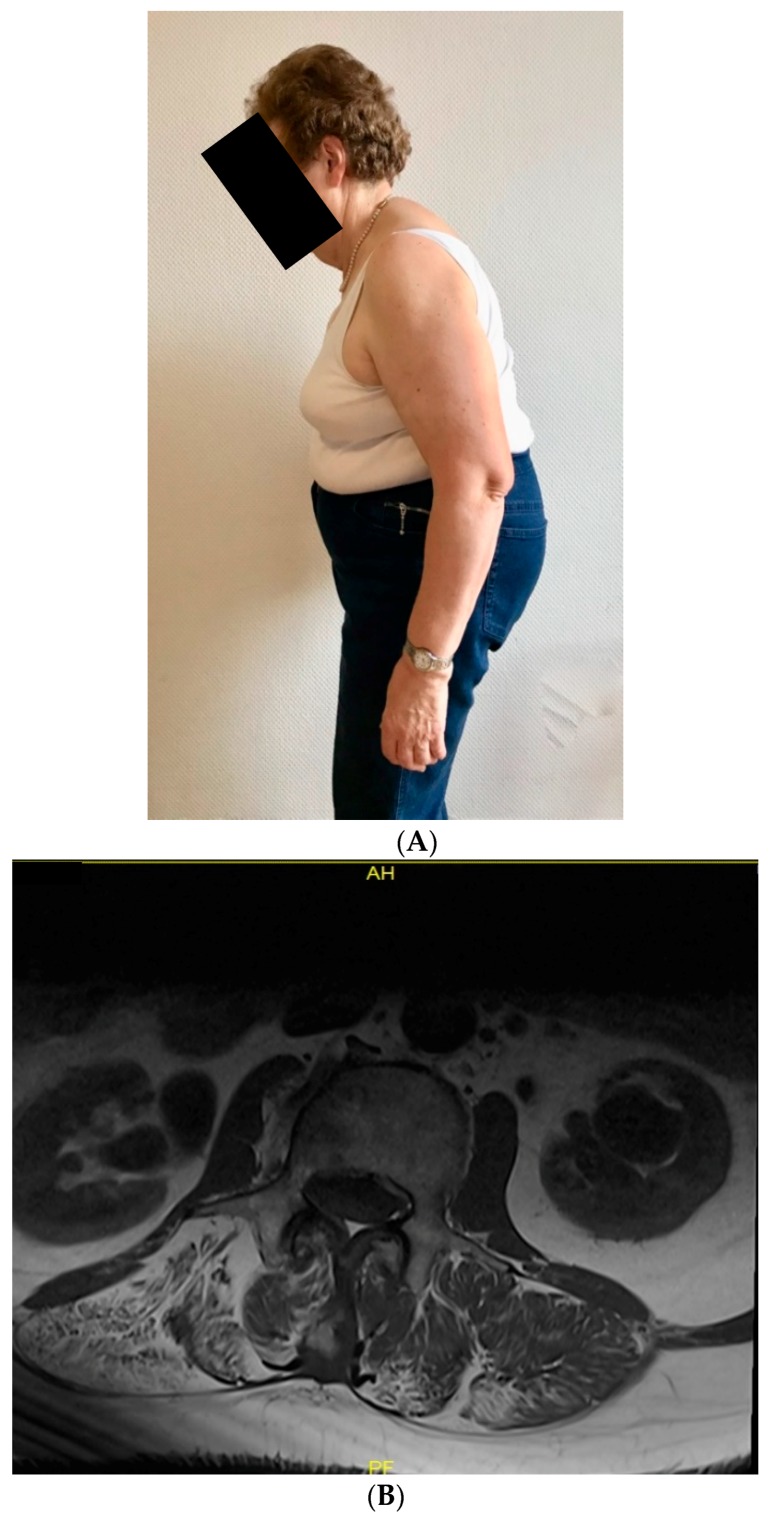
(A) Camptocormia in the patient as the main clinical symptom: upright standing is only possible with bended knees, indicating the severity of camptocormia and (B) transversal T1- weighted spinal MRI showing a significant fatty degeneration of the paravertebral lumbar spine muscles.
